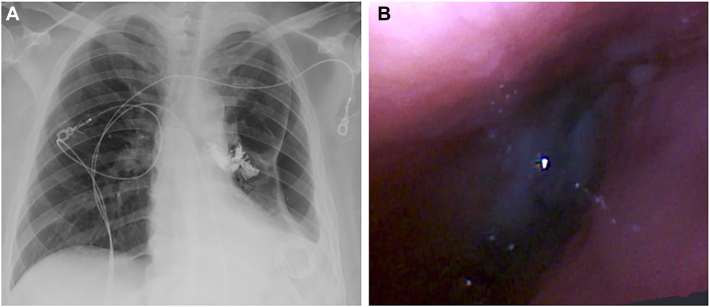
(A) Chest roentgenogram shows a pigtail catheter in a large pleural space with partial upper lobe collapse. (B) Fully expanded upper lobe on a postoperative chest roentgenogram with decreased size residual pleural space.
